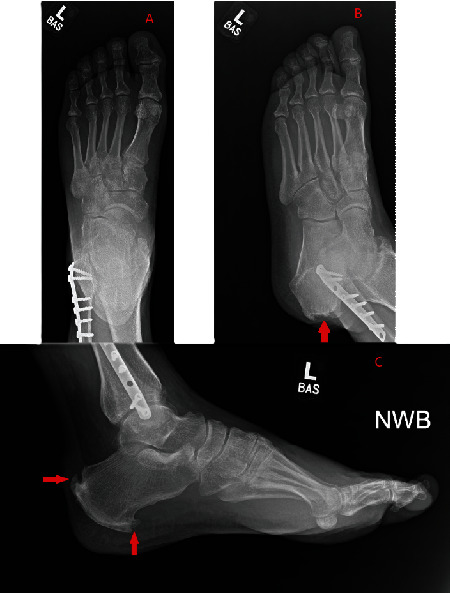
Preoperative radiographs of the left ankle demonstrating anteroposterior (A), oblique (B), and lateral (C) views. Plantar and posterior enthesophytes of the calcaneus are indicated with the arrows.
